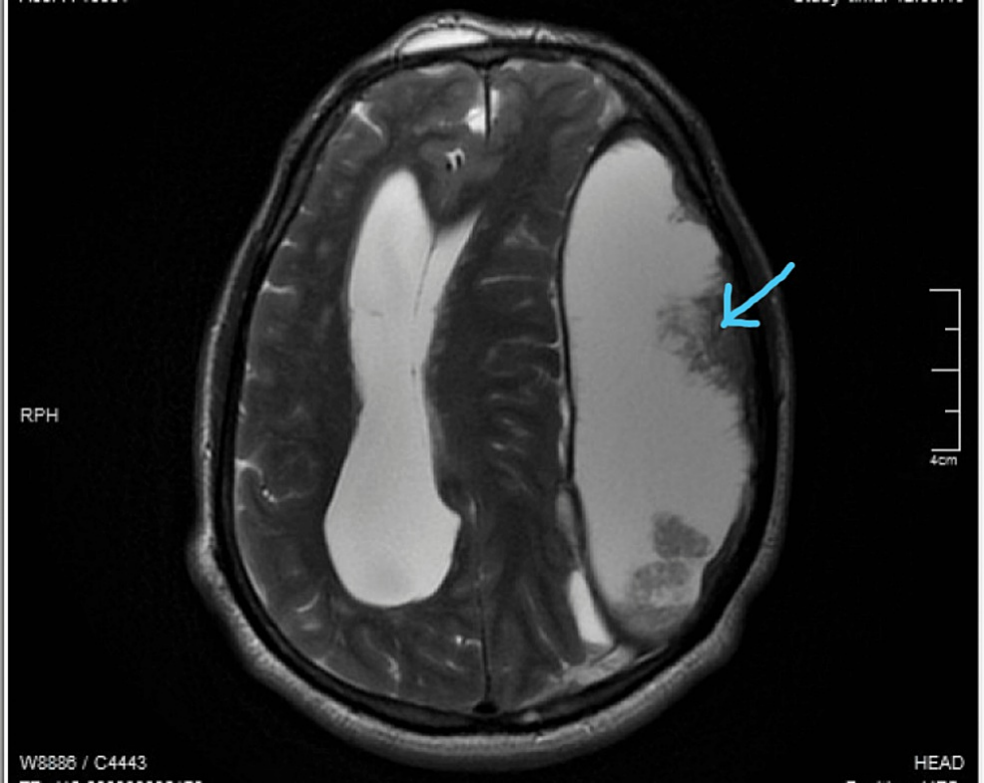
T2 weighted MRI showing a left frontotemporal hematoma with sub-acute changes Arrow indicates the sub-acute change on the left frontotemporal hematoma.
